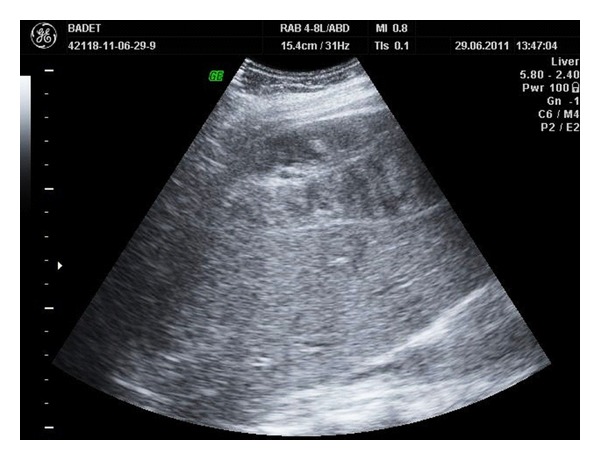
Liver ultrasound reveals large subcapsular hematoma.
